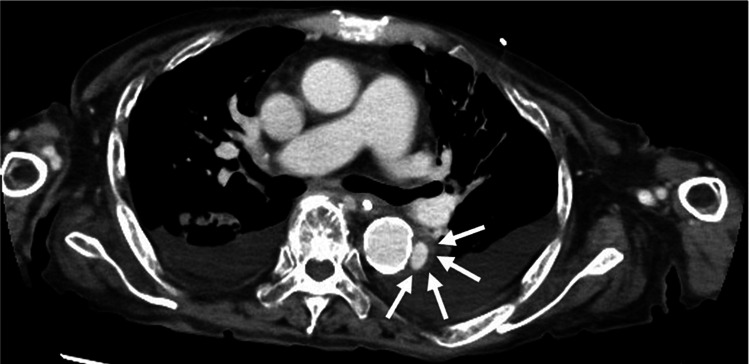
Contrast-enhanced computed tomography of the chest. The white arrows show a localized descending aortic dissecting aneurysm.
